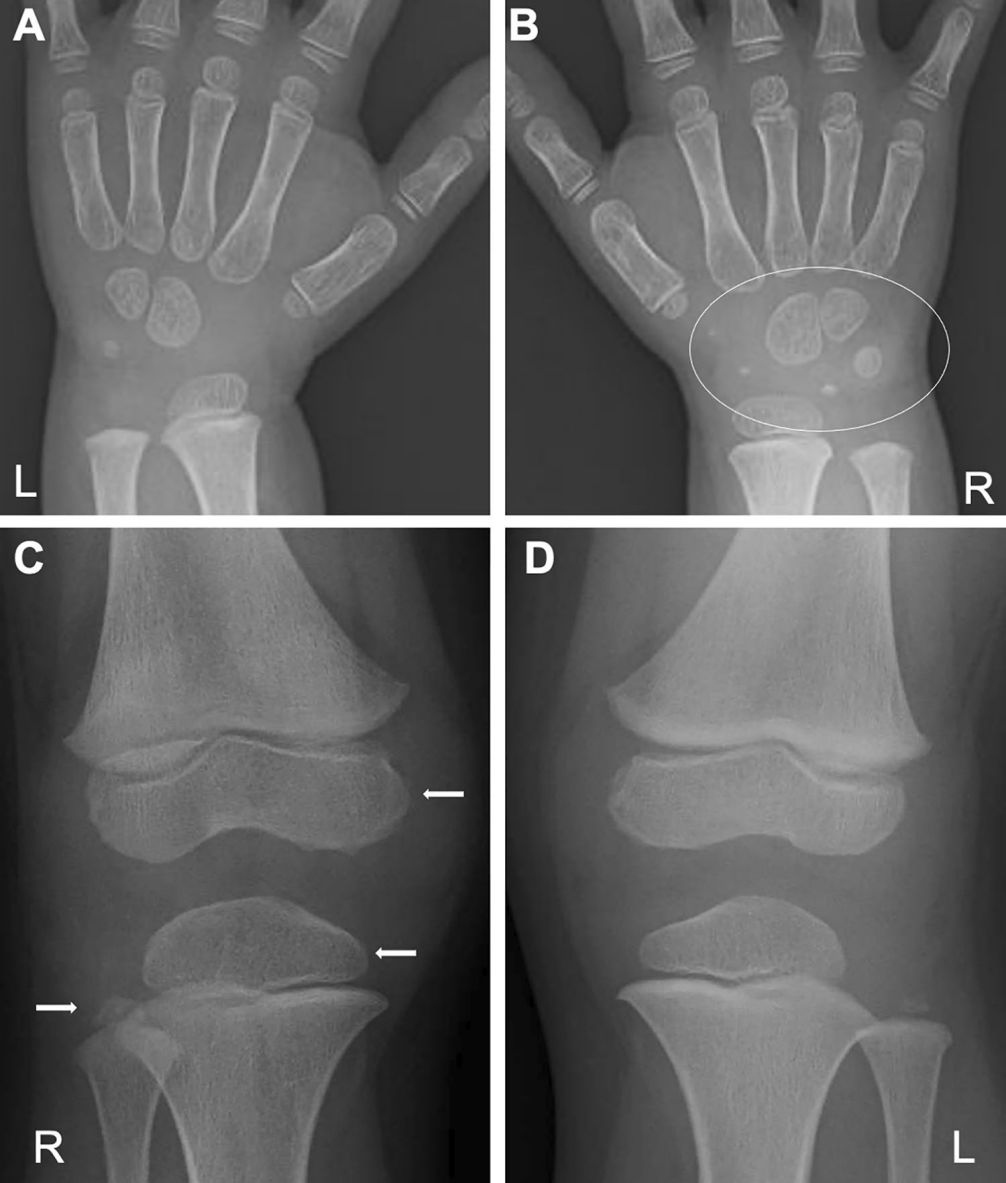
Two cases with oligoarticular type JIA. A-B. A 2-year-old girl with restricted dorsiflexion of the right wrist. The frontal radiographs showed accelerated carpal ossification of the right (circle, B) and a normal left hand (A). C–D. A 3-year-old boy with painful right knee. The frontal radiographs showed large epiphyses (arrows, C) and juxtaarticular osteopenia of the right knee and a normal left knee (D)
